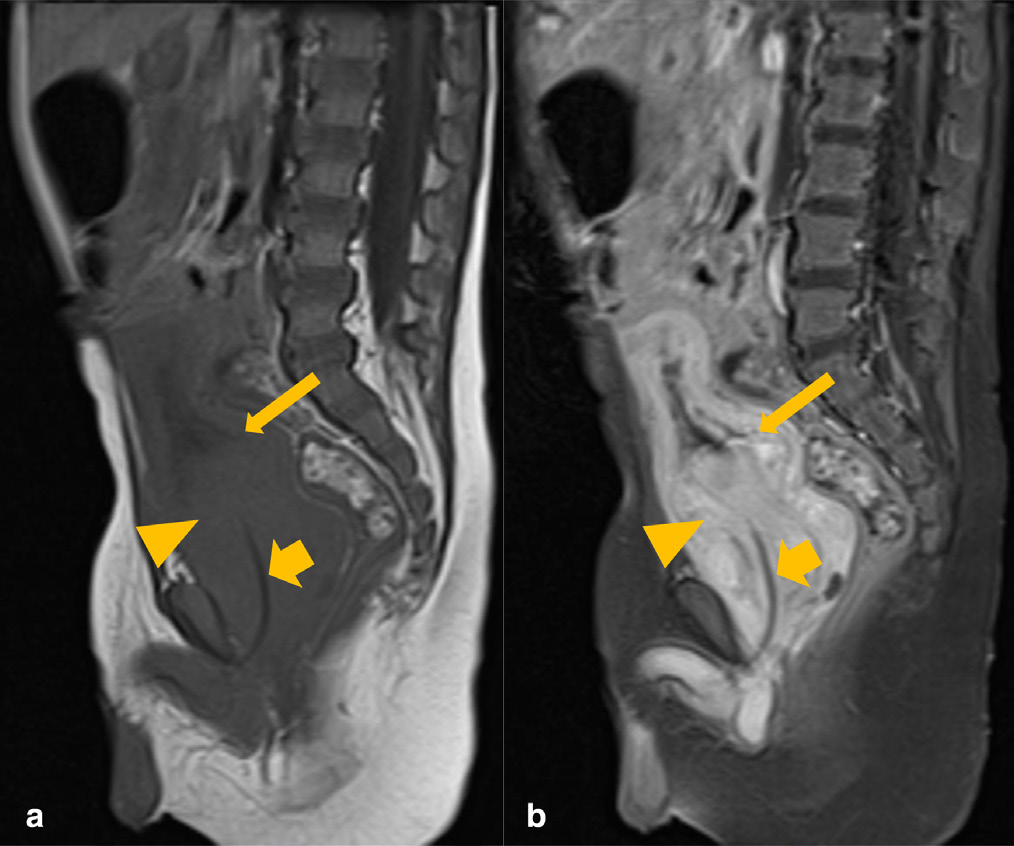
Sagittal T1 pre- (A) and post-contrast (B) MRI images of the abdomen and pelvis. There is an avidly enhancing prostatic mass (orange arrowhead), invaginating into the urinary bladder base (thin orange arrow). The urinary catheter is coursing through the mass (thick orange arrow).
